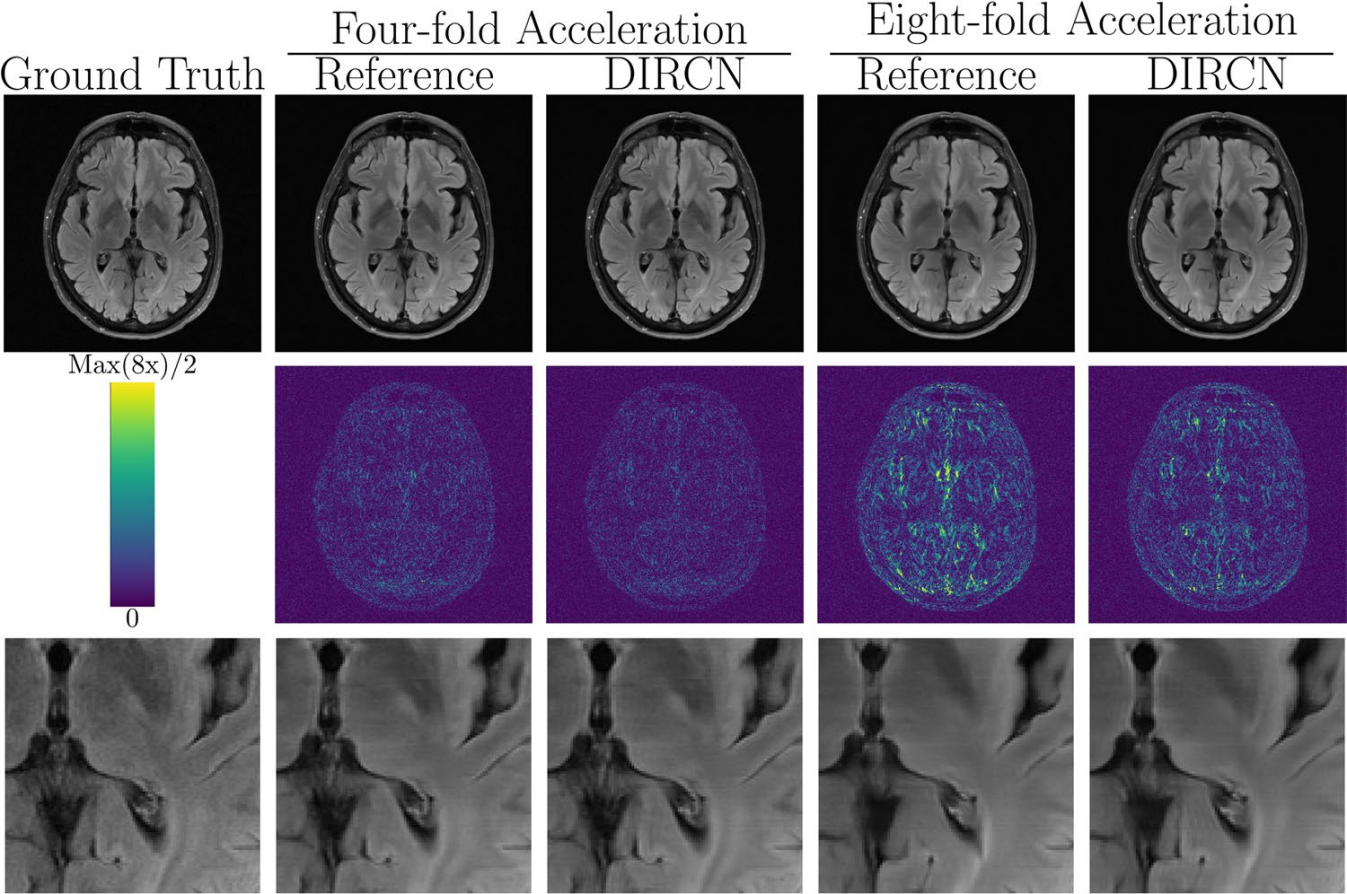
A representative example of a FLAIR reconstruction with the reference model and DIRCN for four- and eightfold acceleration. This includes their respective reconstructions and the corresponding error map (absolute difference) between the fully sampled image and the reconstruction. The colormap goes between 0 and half the maximum error for eightfold acceleration to emphasize visual difference. The bottom images are a region of interest where one can see an erroneous reconstruction for eightfold acceleration for both models
